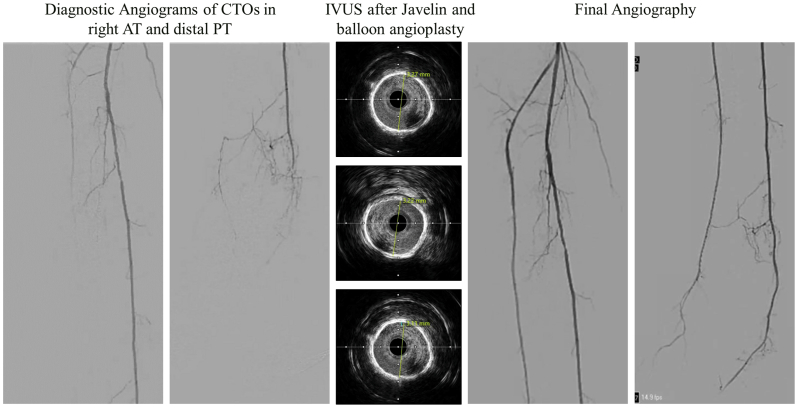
Javelin intravascular lithotripsy (IVL) used to cross and treat calcified chronic total occlusions (*CTOs*) in the right anterior tibial (*AT*) and distal posterior tibial (*PT*) arteries. *IVUS*, Intravascular ultrasound.
